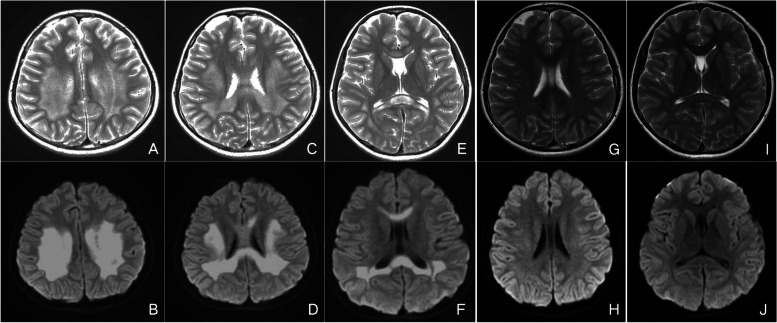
Cranial MRI of case 1 in 2016. Cranial MRI on day 2 showed lesions in the entire corpus callosum and diffuse white matter with moderate hyper intensity on T2-weighted images (A,C,E) and marked hyperintensity on DWI (B,D,F). Follow-up MRI on day 12 showed resolution of the lesions on T2 (G,I) and DWI (H,J)
